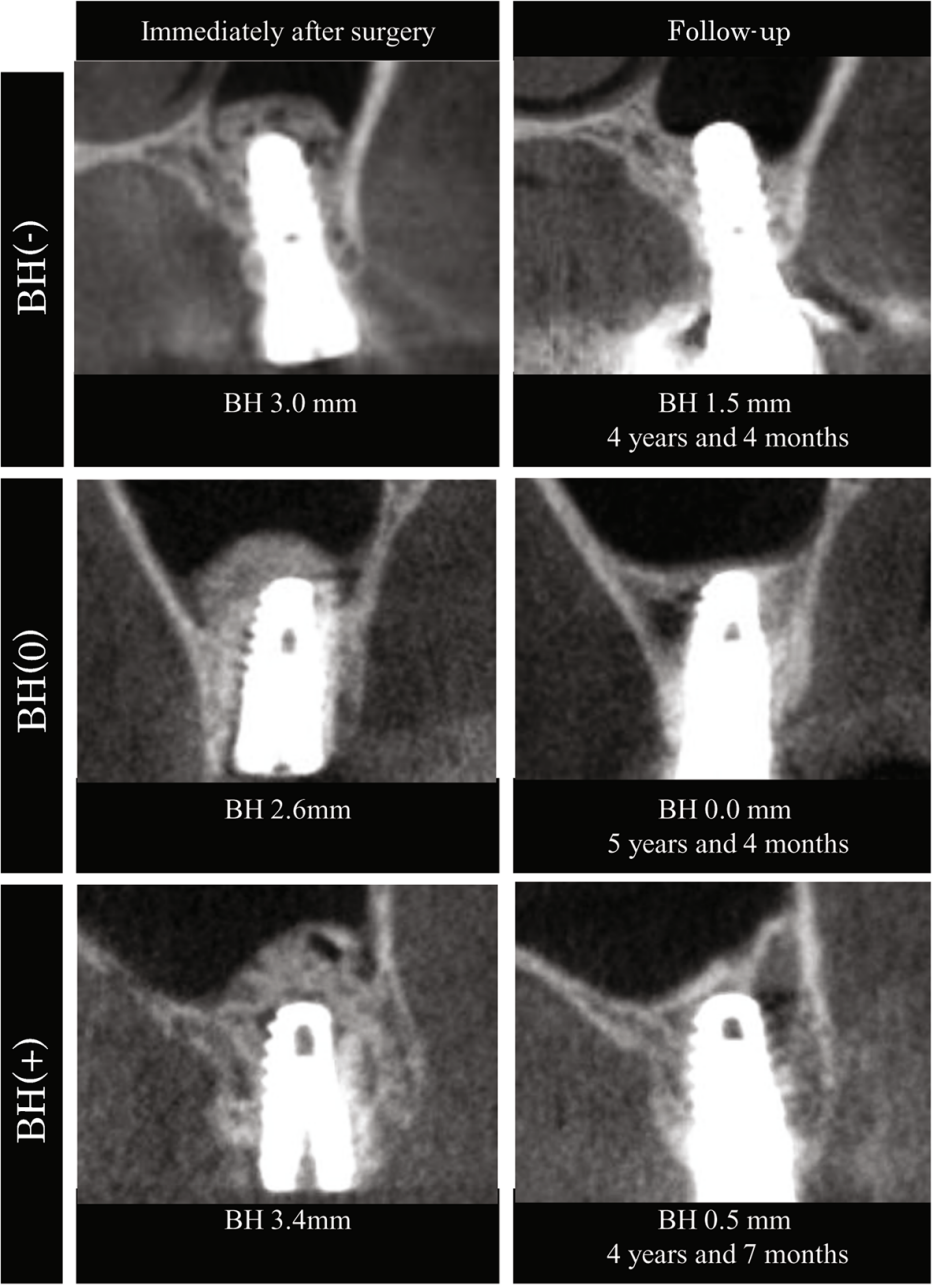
CBCT images immediately after surgery and at follow-up. Immediately after surgery, the maxillary sinus floor was above the implant apex of all implants. At follow-up, the maxillary sinus floor was below the implant apex (BH < 0), at the same height (BH = 0), or above implant apex (BH > 0). BH, augmented bone above apex of the implant
